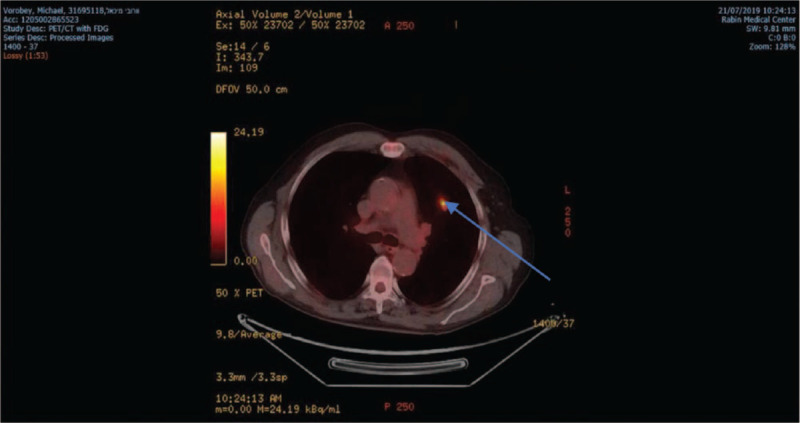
FDG uptake in left upper lobe on PET CT.
